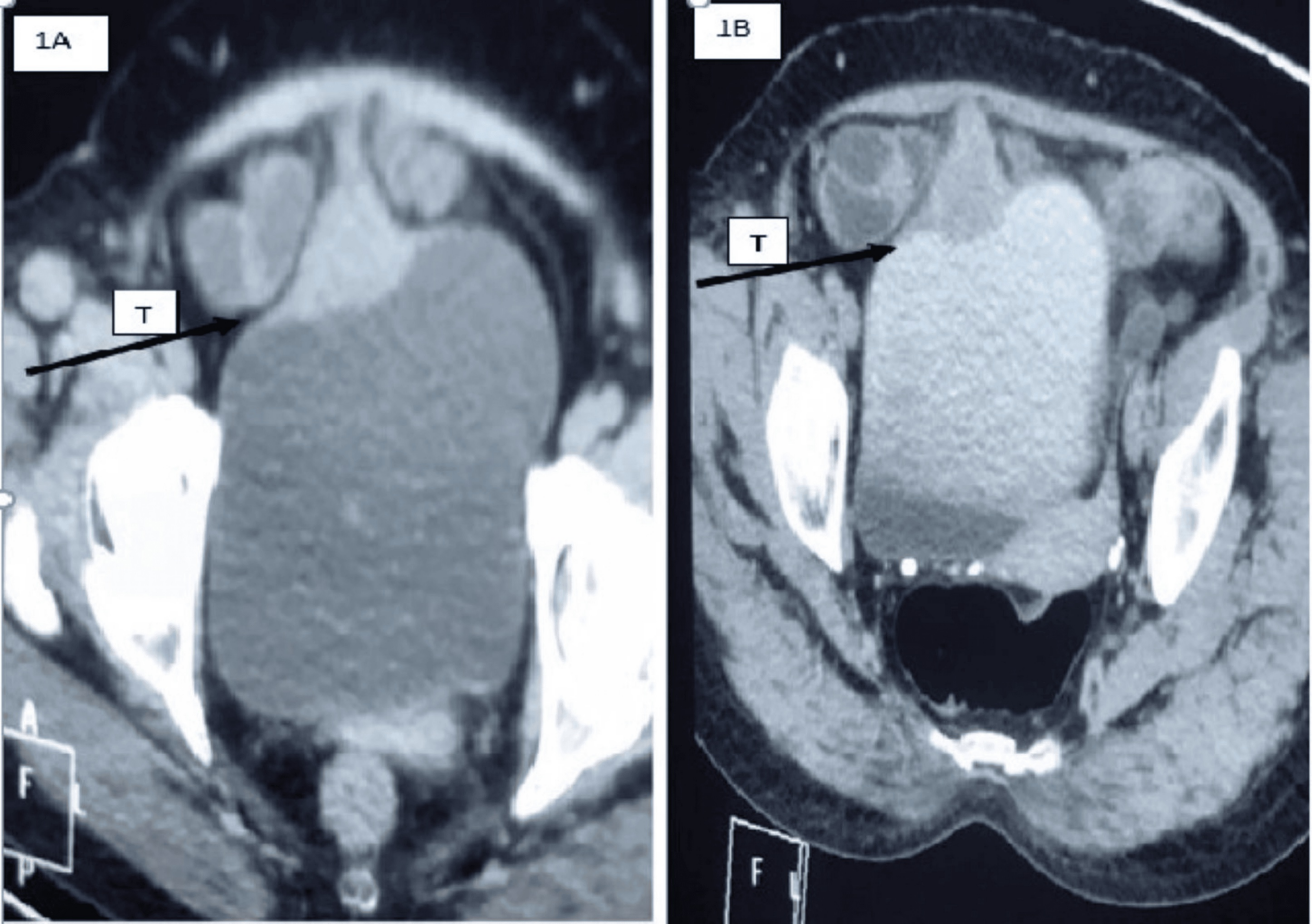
Contrast-enhanced CT images showing urachal growth 1a and 1b depicting plain and CECT images confirming a urachal growth (T) (black arrowhead)
